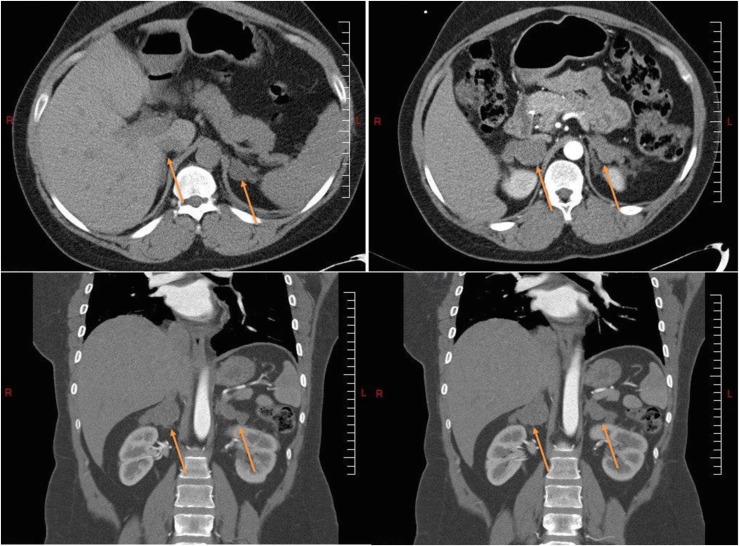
CT images from case 4 showing sagittal and coronal views of bilateral macronodular hyperplasia (arrows).
